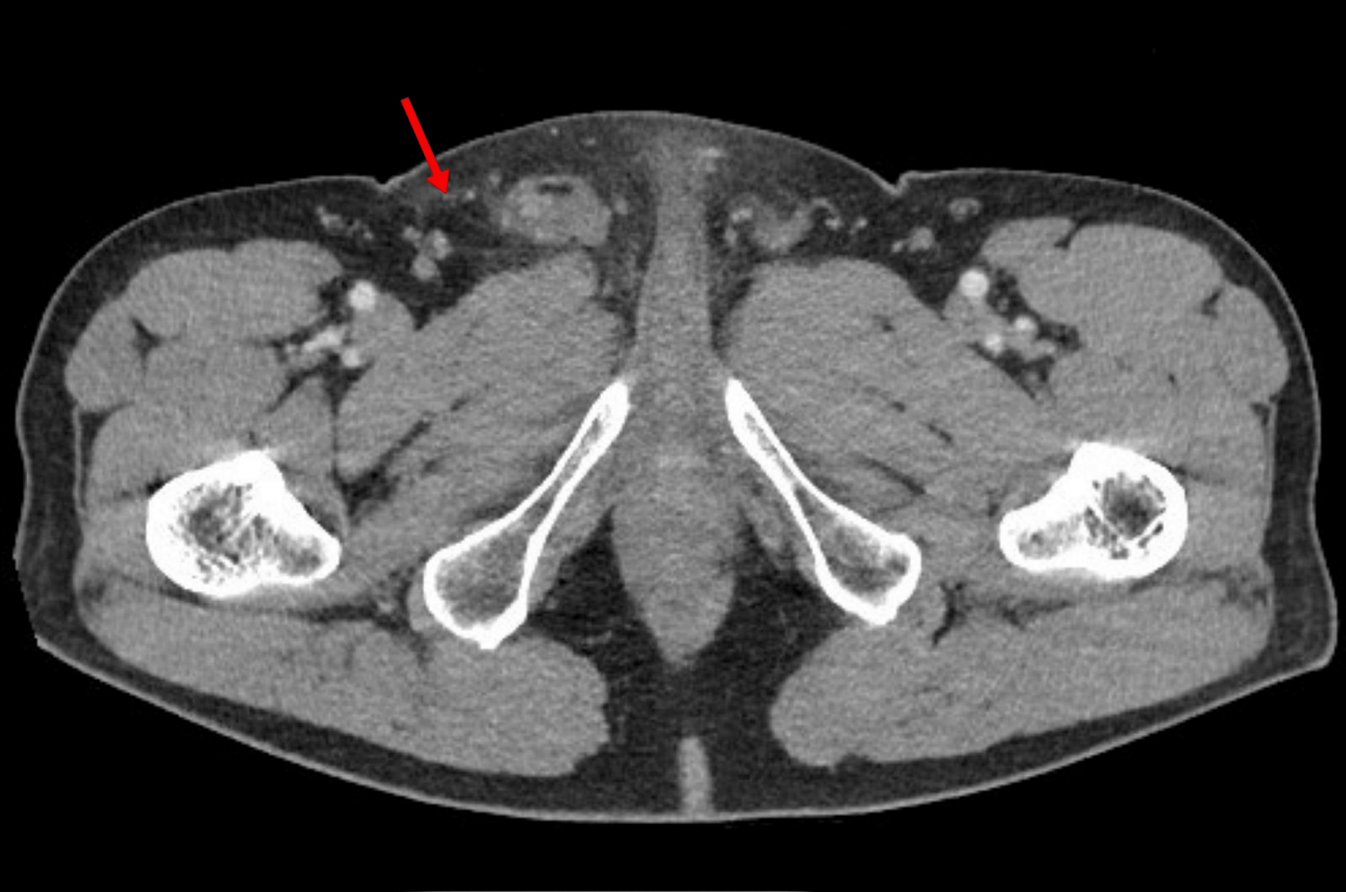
The CT scan shows lower abdominal wall inflammation, as evidenced by fat stranding labeled with an arrow.
